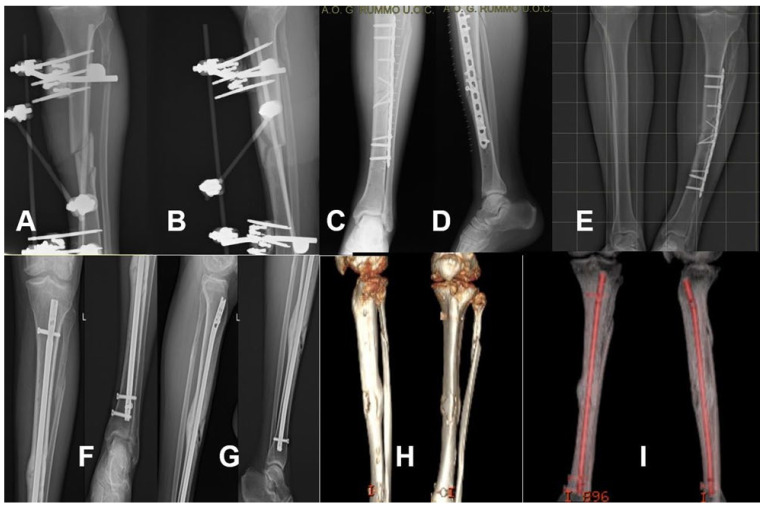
(A,B) A 43-year-old male crashed off his motorbike, open GA IIB tibia fracture, treated with Modular External Fixator in an emergency. (C,D) After 21 days of trauma, the patient underwent tibial plating fixation. (E) One month after surgery, the plate underwent bending deformation, resulting in a tibial varus. (F,G) Three months after the nonunion following nailing surgery with ASFC, the X-Rays showed bone callus on the third cortex. (H,I) At 6 months, the 3D CT scan indicated the perfect creation of RUST.
